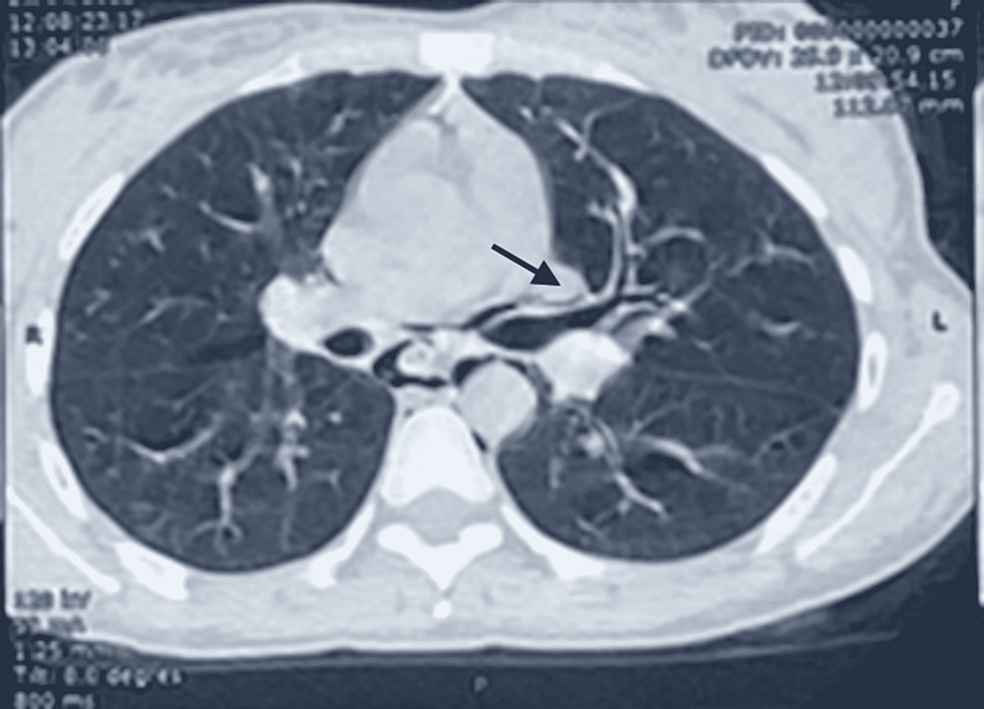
Thoracic CT scan showing the Macklin effect. The CT scan image confirms the Macklin effect by demonstrating air dissection along the peribronchovascular sheaths.
